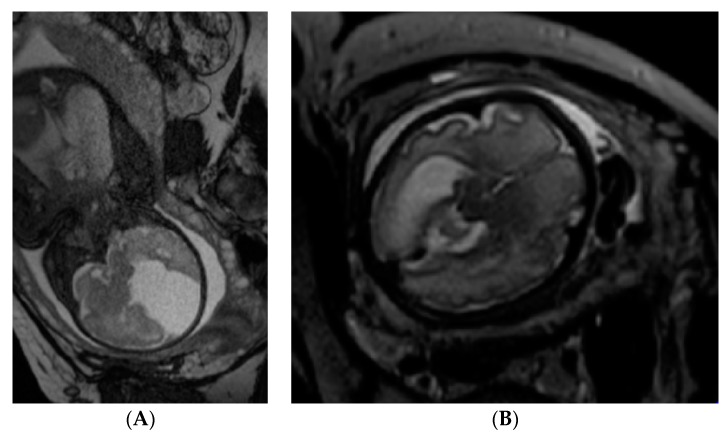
(A,B) Coronal and axial MRI scans of the fetuses brain. Left posterior and temporal horn was dilated—18 mm. From cisterna ambient interhemispheric fissure O-P-F and liquor collections O-P-F 6.7 × 3.3 cm with septum. Signs of corpus callosum agenesis.
